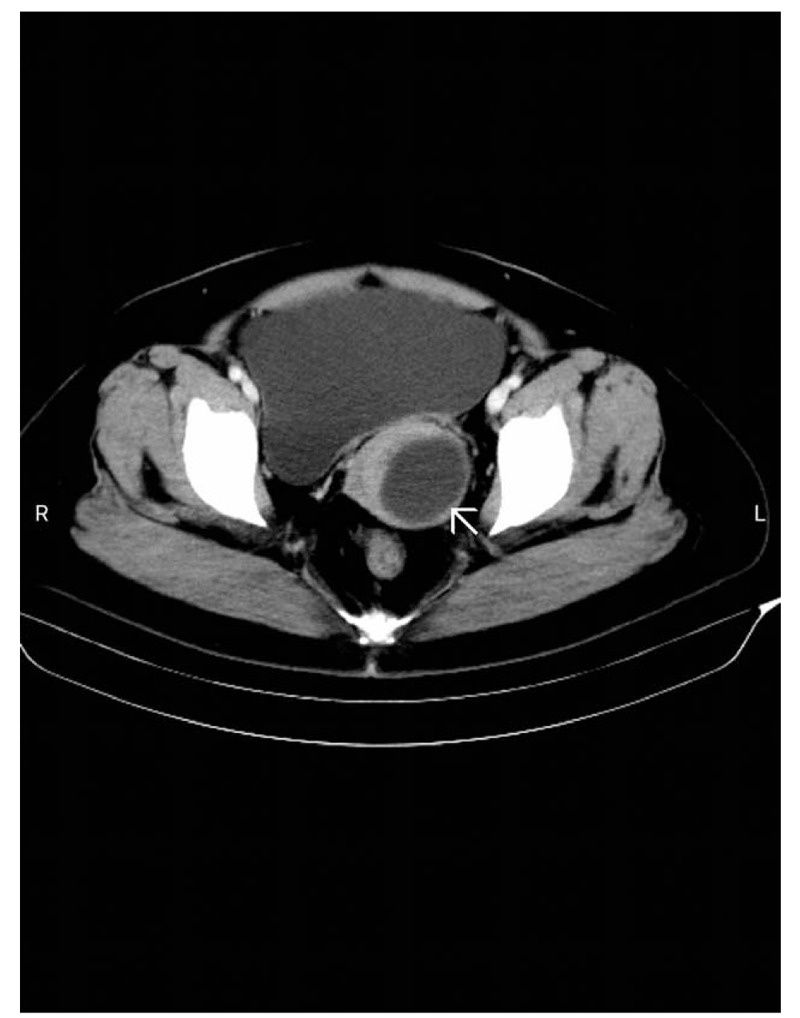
Pelvic computed tomography scan showing an enlarged and distended bladder and a cervical cyst (arrow).
